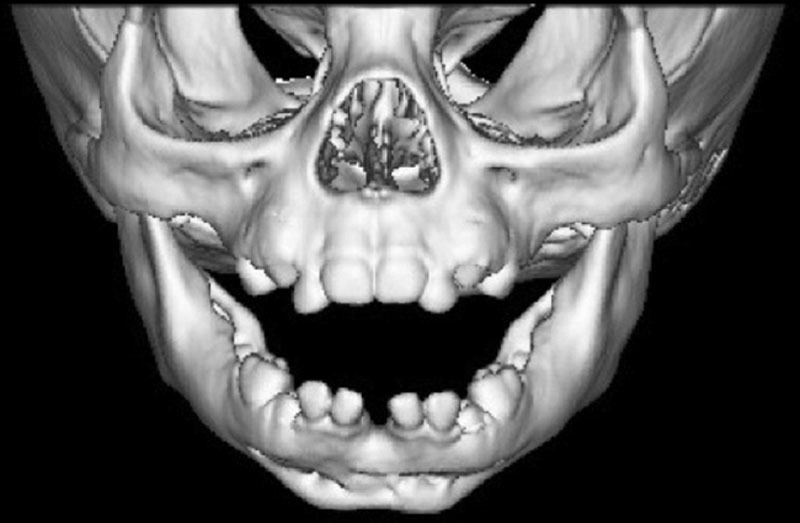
Postoperative computed tomography scan of face demonstrating union at mandible symphysis.
